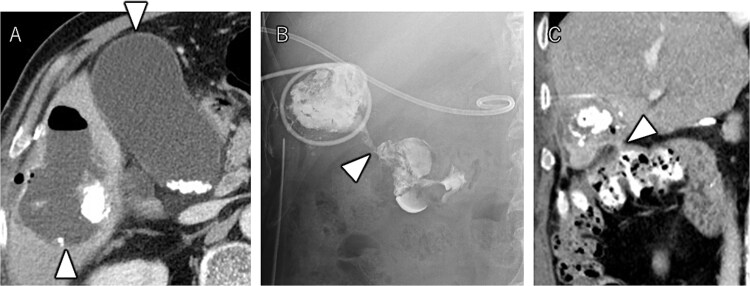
CT demonstrating a fluid collection with air inside the liver tumor, as well as enlargement and wall thickening of the gallbladder (A). Fistulography performing through the liver abscess drainage catheter revealed contrast filling in the colon (B). Subsequent CT scan showed that the liver tumor was adjacent to the catheter, with opacification of the colonic lumen (C).
